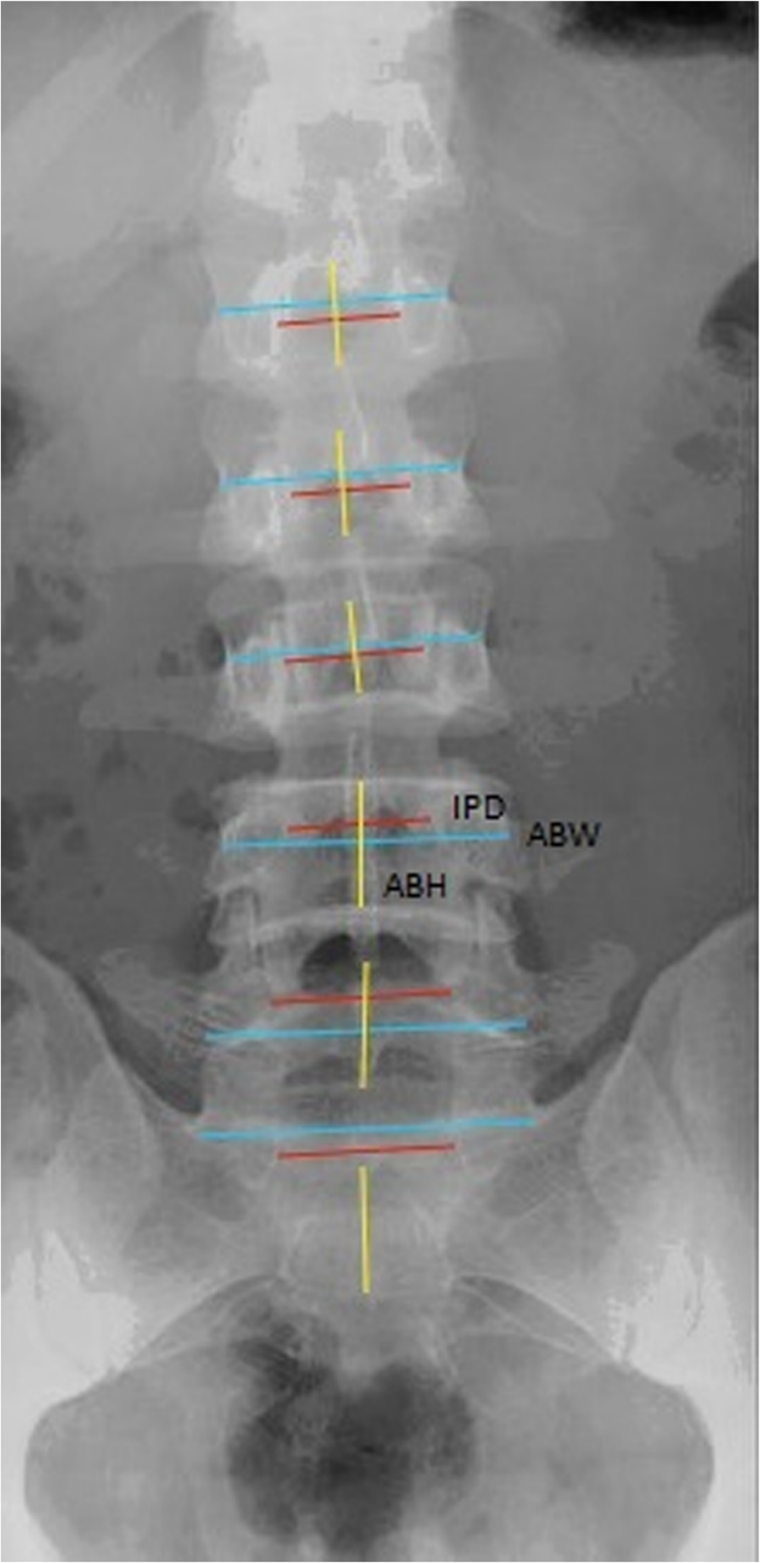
Measurement scheme for the anteroposterior standing radiograph: axial vertebral body width (*ABW: light blue*), axial vertebral body height (*ABH: yellow*), and interpedicular distance (*IPD: red*)
